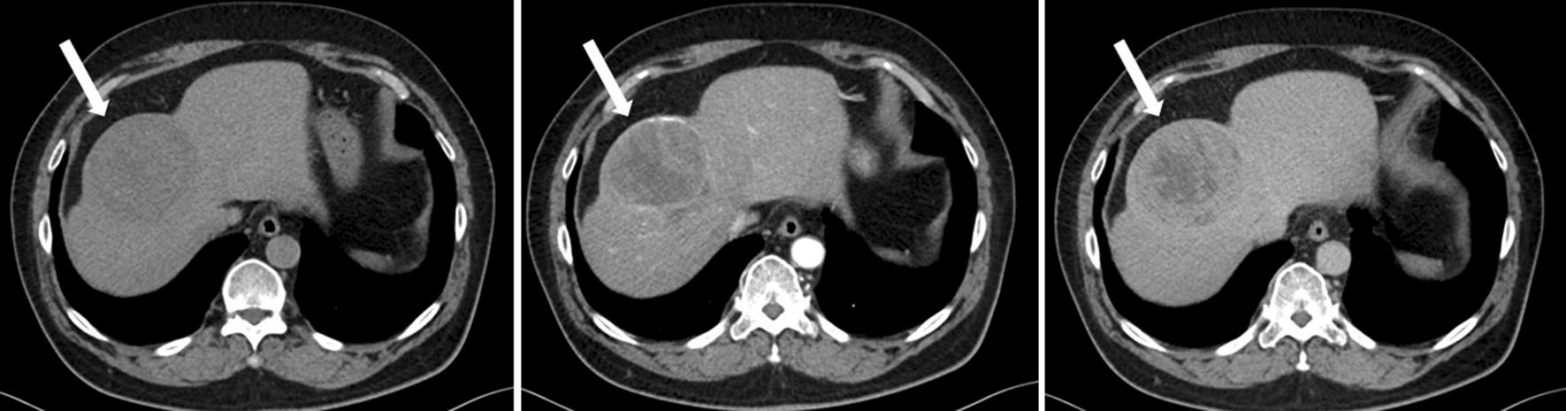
Abdominal computed tomography (CT) images. Contrast-enhanced computed tomography revealed a tumor in segment 8 of the liver. The tumor was 80 mm in diameter and was adjacent to the middle hepatic vein. The white arrow indicates the tumor. Left: plain; middle: arterial phase; right: portal or delayed phase
